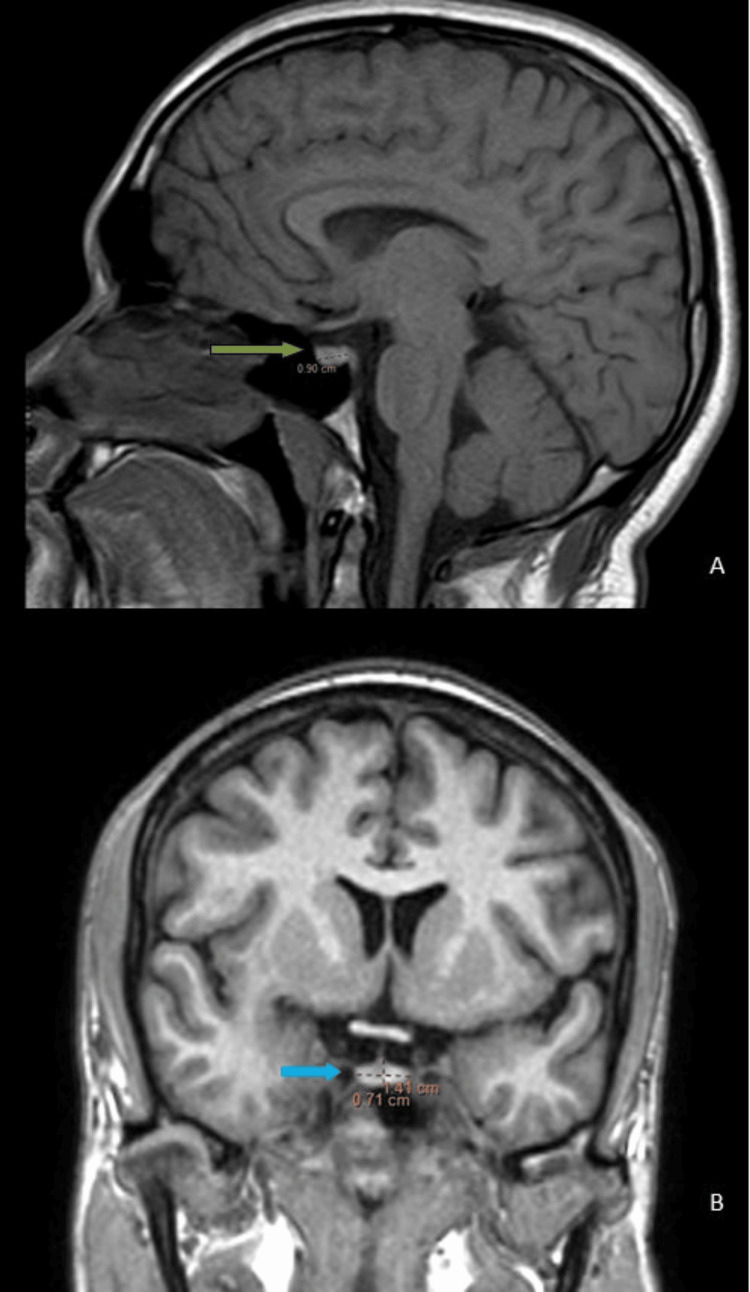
(A, B): MRI of the sella turcica. (A) T1 mid-sagittal: the pituitary gland in a 17-year-old male shows an anteroposterior of 9 mm (green arrow). (B) T1 mid-coronal: the pituitary gland of the same male shows a transverse dimension and height of 14 mm and 7 mm, respectively (blue arrow). The pituitary gland volume is 441 mm3
